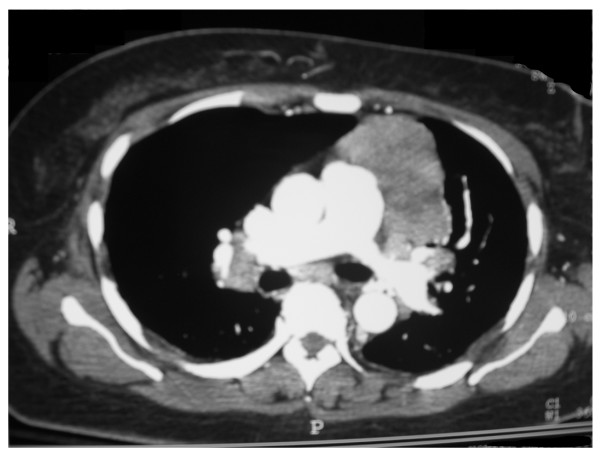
CT chest image revealing large necrotic mass in the left anterior mediastinum and bilateral hilar lymphadenopathy.
